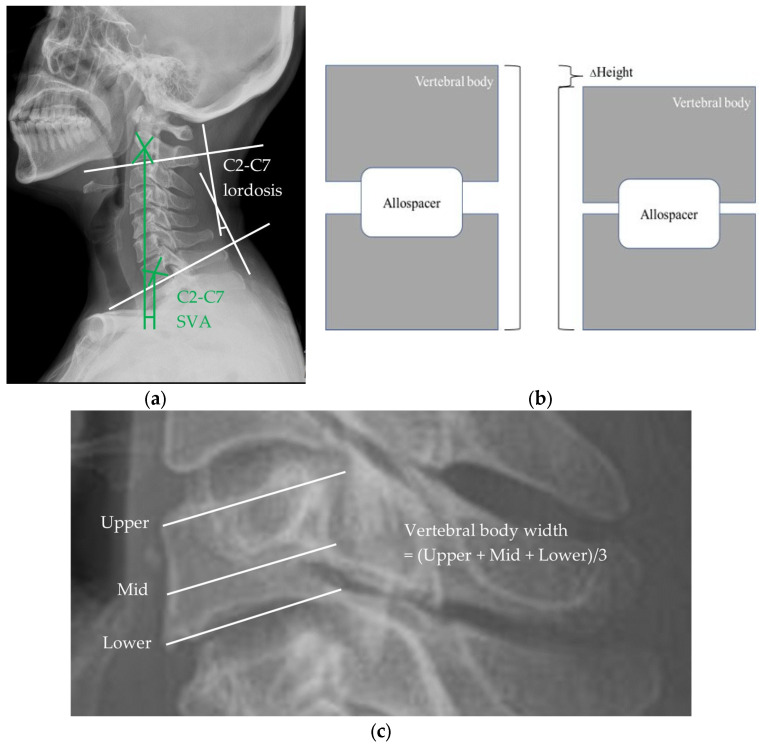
Measurements of parameters on lateral cervical radiograph: (a) C2–7 cervical lordosis (CL) and C2–7 sagittal vertical axis (SVA); (b) vertical height change; (c) and vertebral body width schemes following the same formatting.
